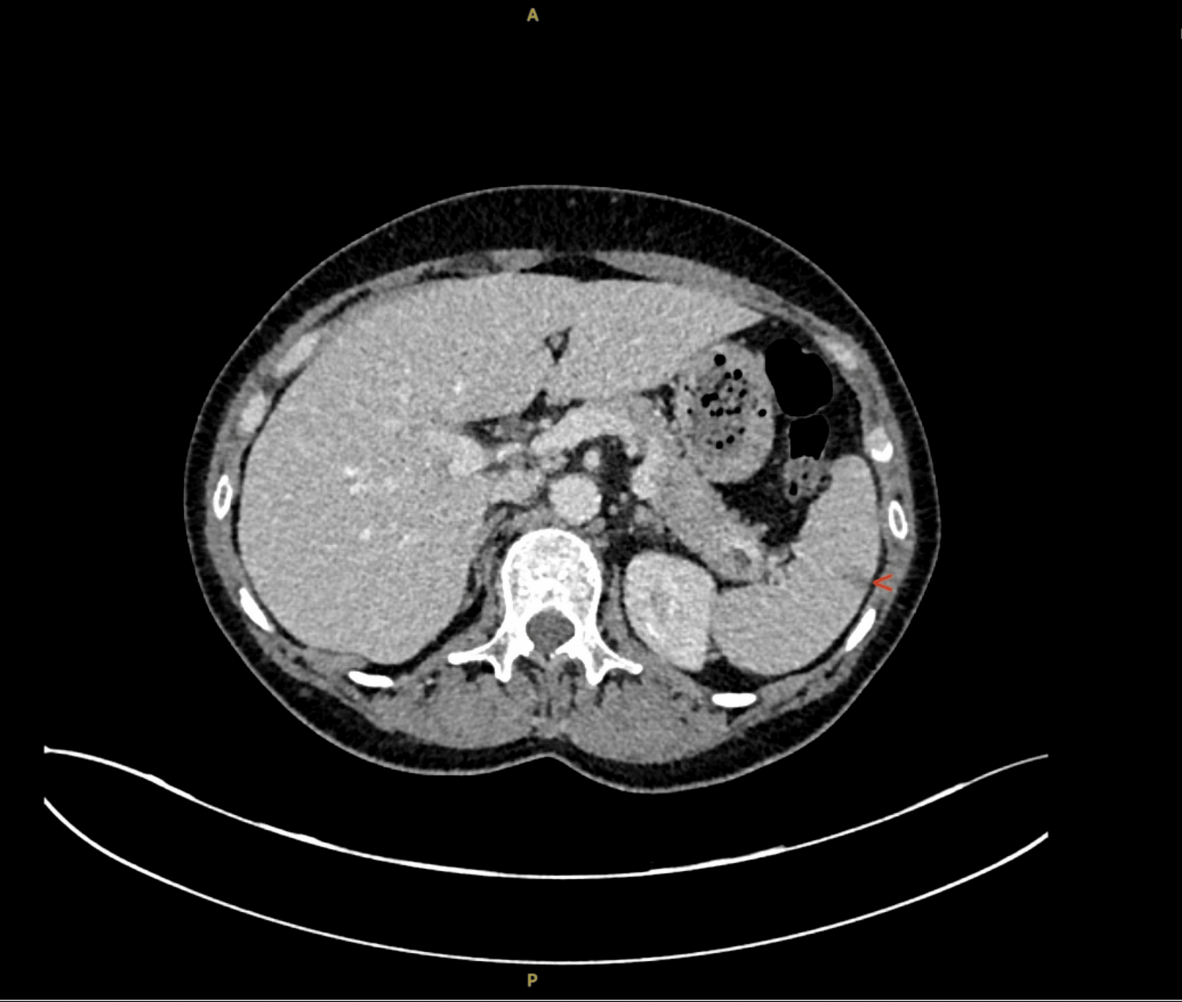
CT abdomen-pelvis showing linear peripheral hypodensity of spleen
